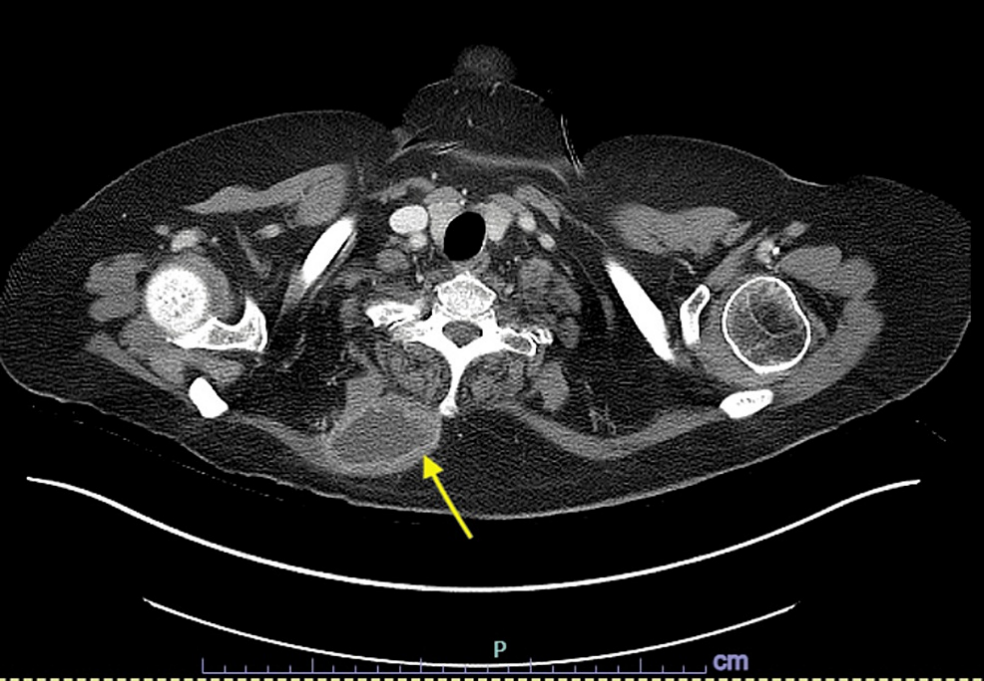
Computed tomography (CT) of the chest showed a soft tissue mass The patient's CT of the chest with contrast showed a right paraspinal abscess (yellow arrow) measuring 5.5 x 3.8 x 5.5 cm
